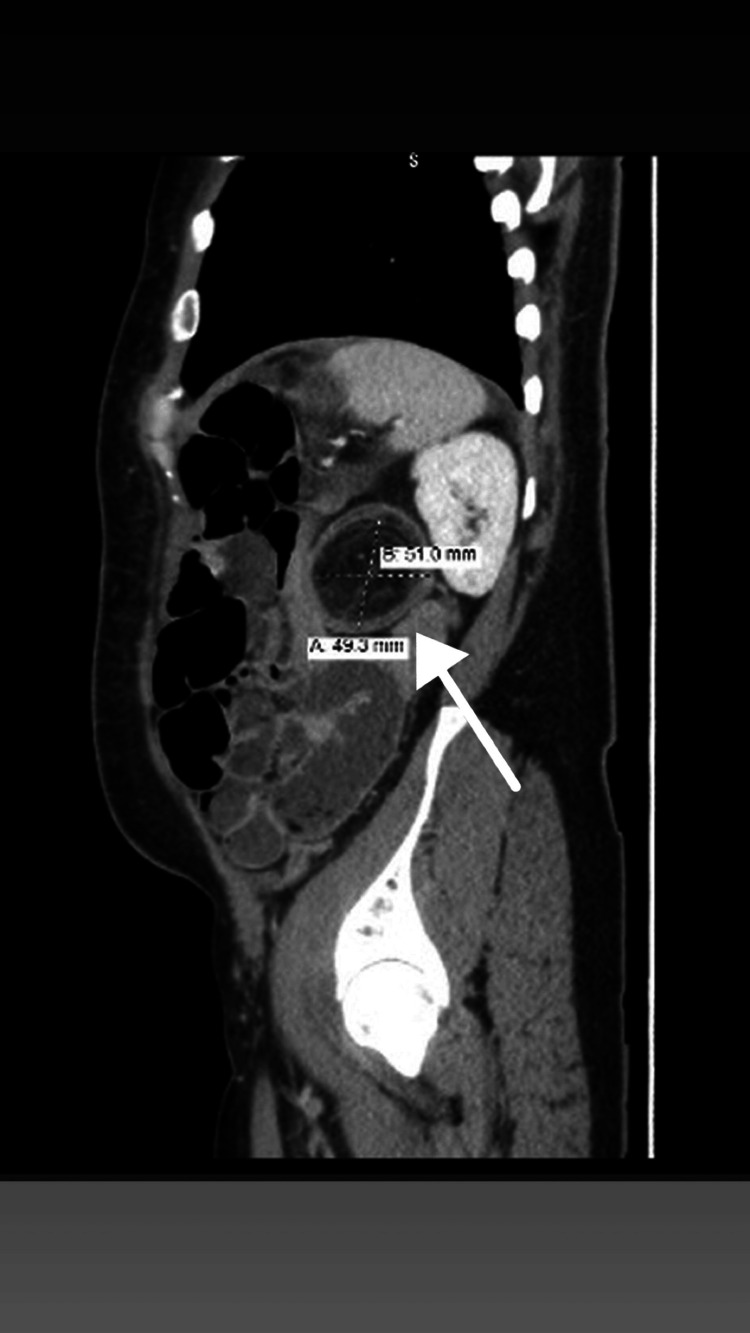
Sagittal CT showing features of partial small bowel obstruction The distal ileum is mildly dilated (up to 3.7 cm) with an element of the small bowel feces sign, suggesting partial obstruction. The invaginated colonic segment demonstrates decreased enhancement as it passes through the intussusception. No pneumatosis is noted
